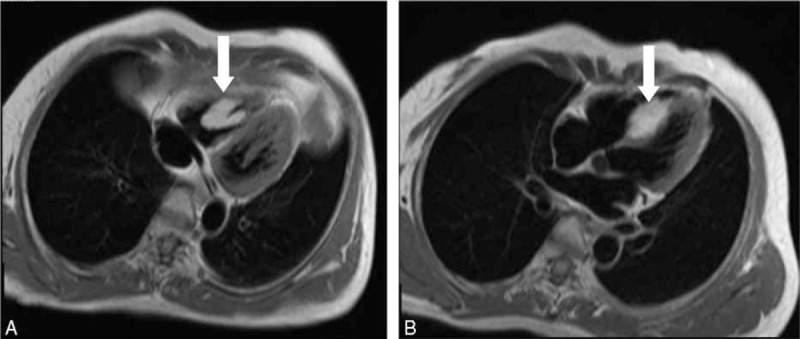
An echogenic RV mass is seen on transthoracic echocardiography. The horizontal, long-axis (4-chamber view), T1-weighted, black-blood image showing a well-circumscribed, high-signal mass in the RV cavity (arrow, A). The horizontal, long-axis, T1-weighted black-blood image showing the mass adherent to IVS (arrow, B). IVS = interventricular septum; LA = left atrium; LV = left ventricle; RA = right atrium; RV = right ventricle.
